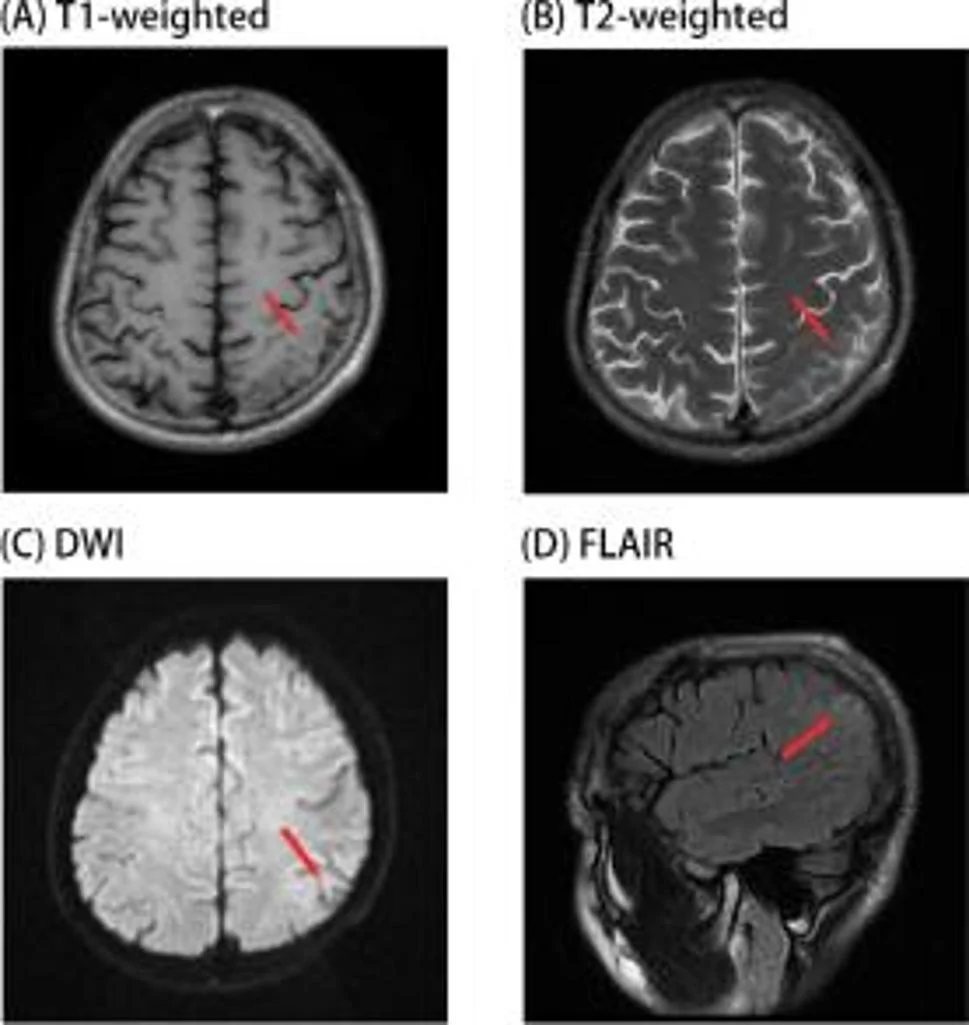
Follow-up Brain Magnetic Resonance Imaging (MRI) Findings. Follow-up brain MRI (A/B/C/D) after 1.5 months of entecavir therapy shows near-complete resolution of the lesion (arrow)
